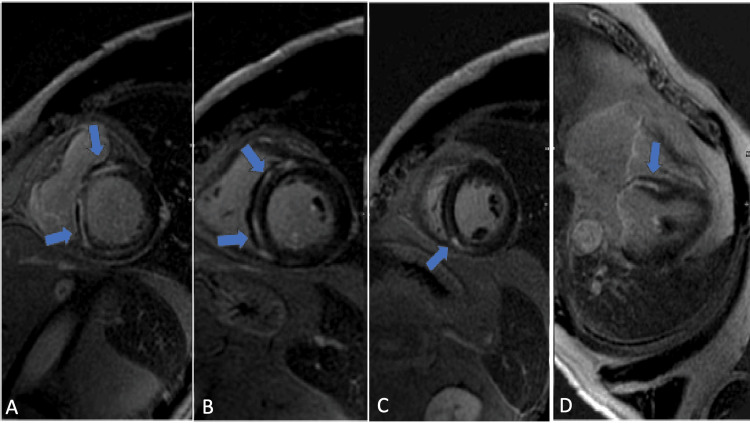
Cardiovascular MRI demonstrates mid-myocardial delayed enhancement (gadolinium) involving the basal anterior (A), anteroseptal, inferoseptal (B), inferior, and inferoseptal segments (C), as well as the mid-anteroseptal segment (D) (blue arrows).
